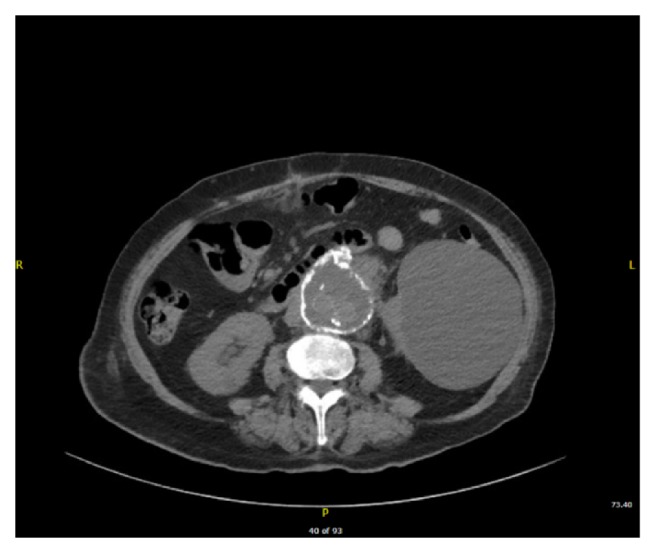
Computed tomography of abdomen showing nonspecific findings, irregularity of the “aneurysmal sac” with a small amount of fluid around the sac.
